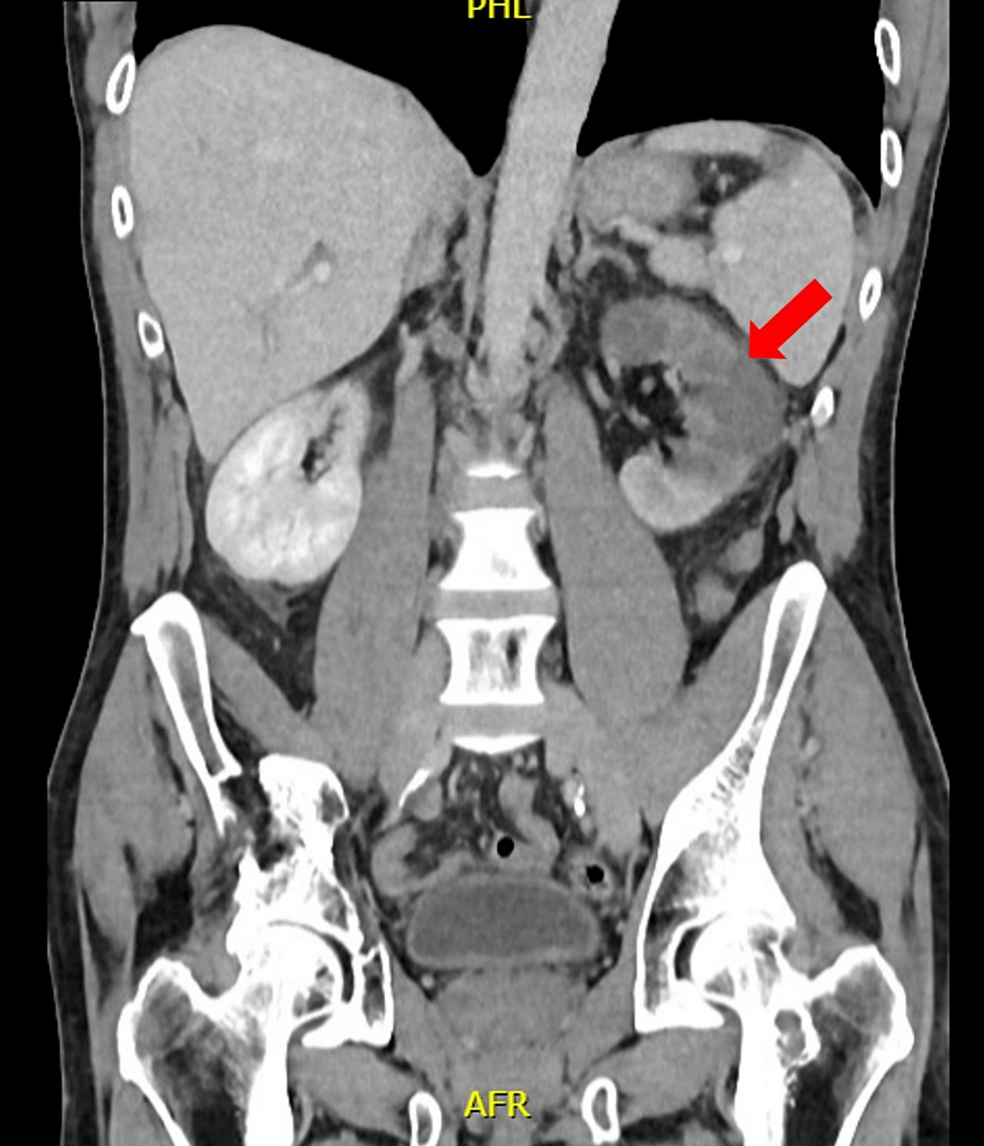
Enhanced CT scan showing a large renal infarction
